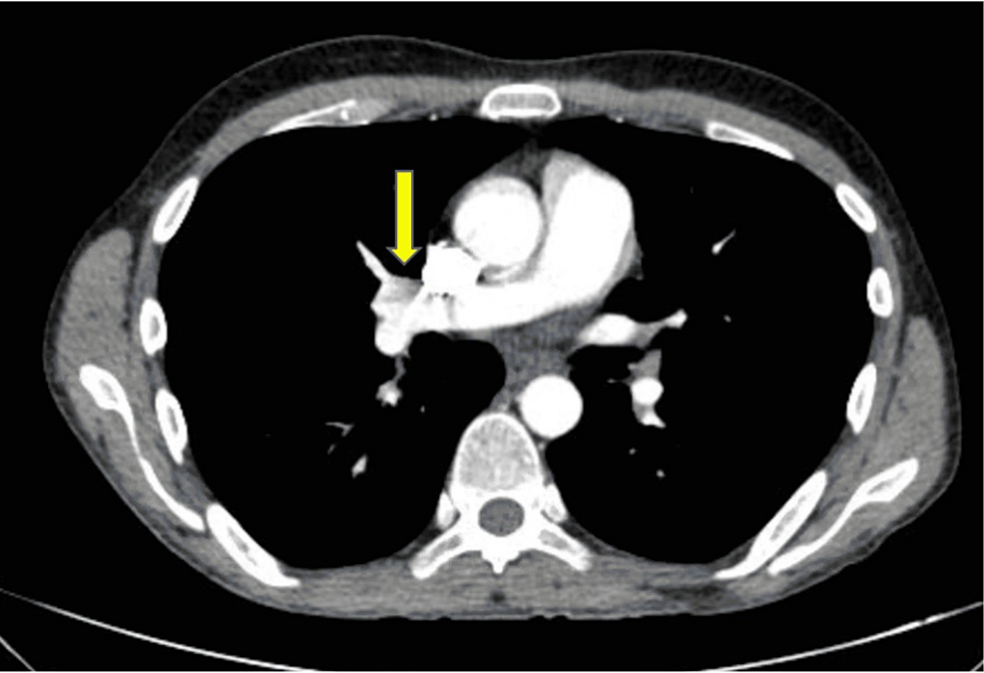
CTA of chest demonstrating right hilar lymphadenopathy (yellow arrow). CTA: Computed tomography angiography.
